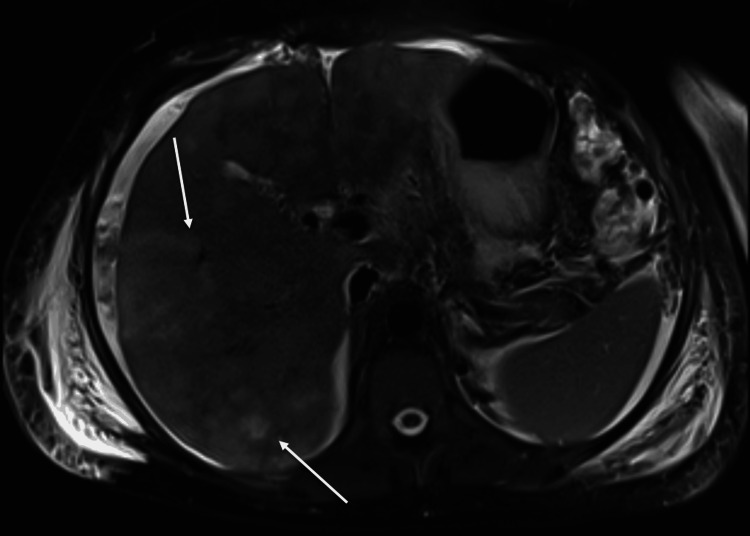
MRI abdomen from case two demonstrating diffuse metastasis to the liver and ascites.
